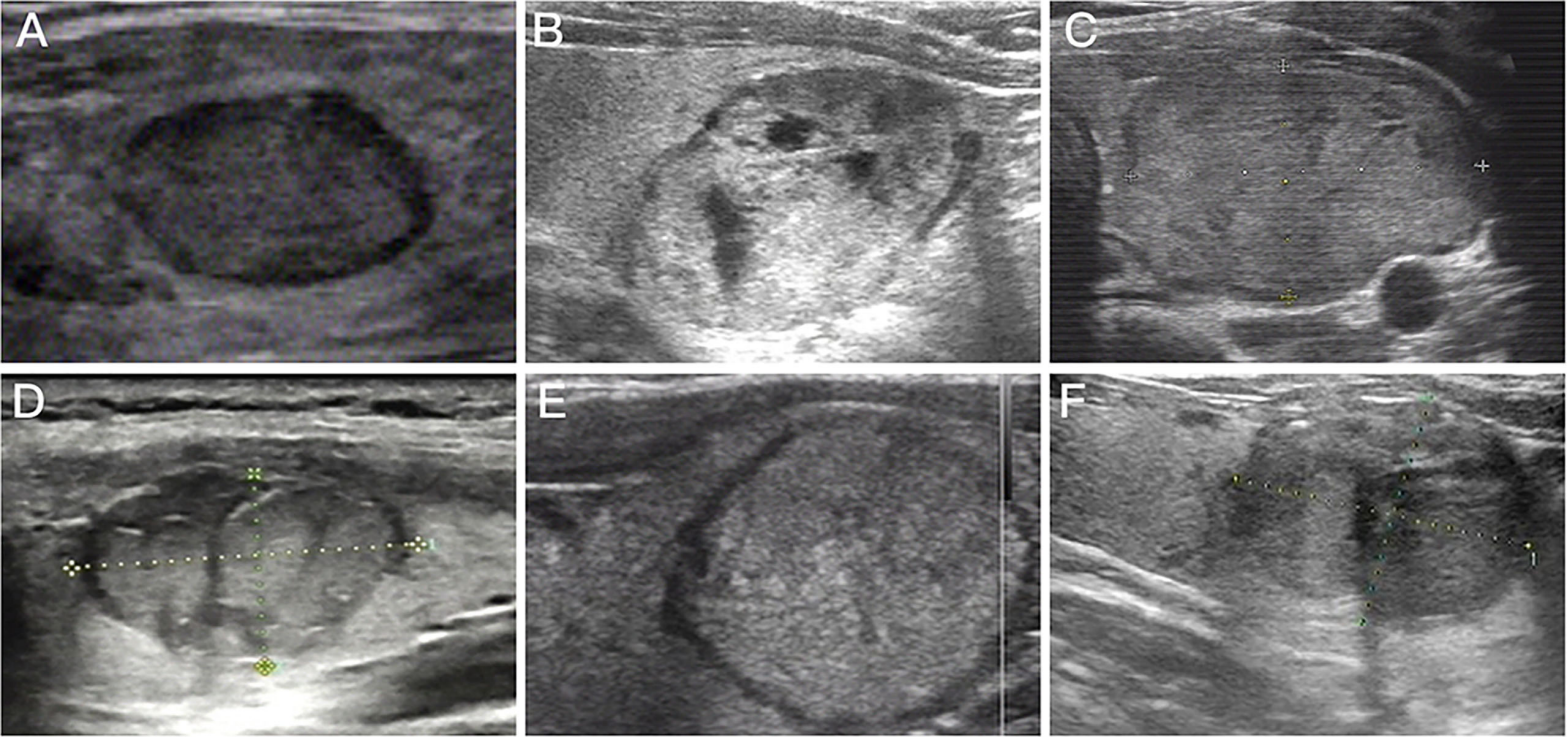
Preoperative ultrasound images for follicular thyroid adenoma and follicular thyroid carcinoma. (A) Preoperative ultrasound image of nodule with regular margin diagnosed as follicular thyroid adenoma; (B) Mixed nodule that postoperative pathological diagnosis was follicular thyroid adenoma; (C) Preoperative ultrasound image of isoechoic nodule diagnosed as follicular thyroid adenoma; (D) Preoperative ultrasound image of nodule with irregular margin diagnosed as follicular thyroid carcinoma; (E) Purely solid nodule that postoperative pathological diagnosis was follicular thyroid carcinoma; (F) Preoperative ultrasound image of hypoechoic nodule diagnosed as follicular thyroid carcinoma.
